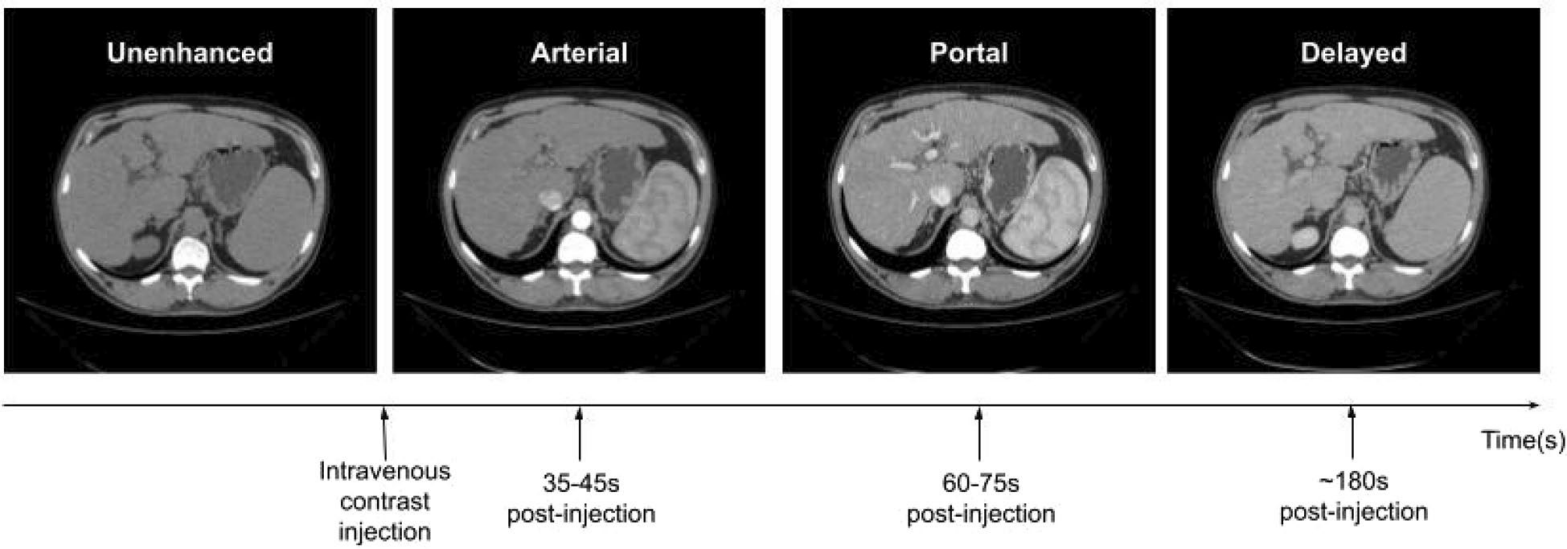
Demonstration of the contrast phases of a liver CT scan.
